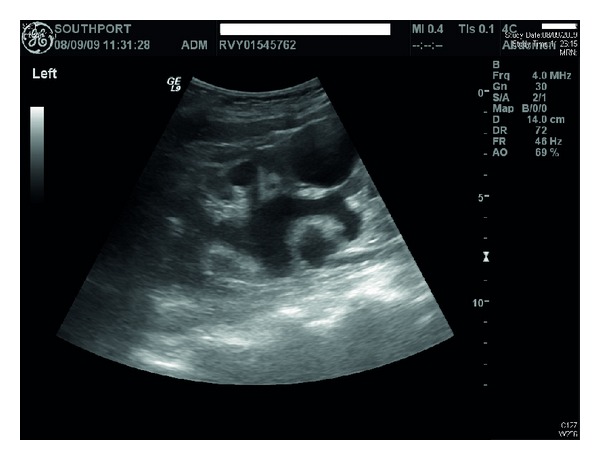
Ultrasound revealed moderate hydronephrosis of left kidney.
